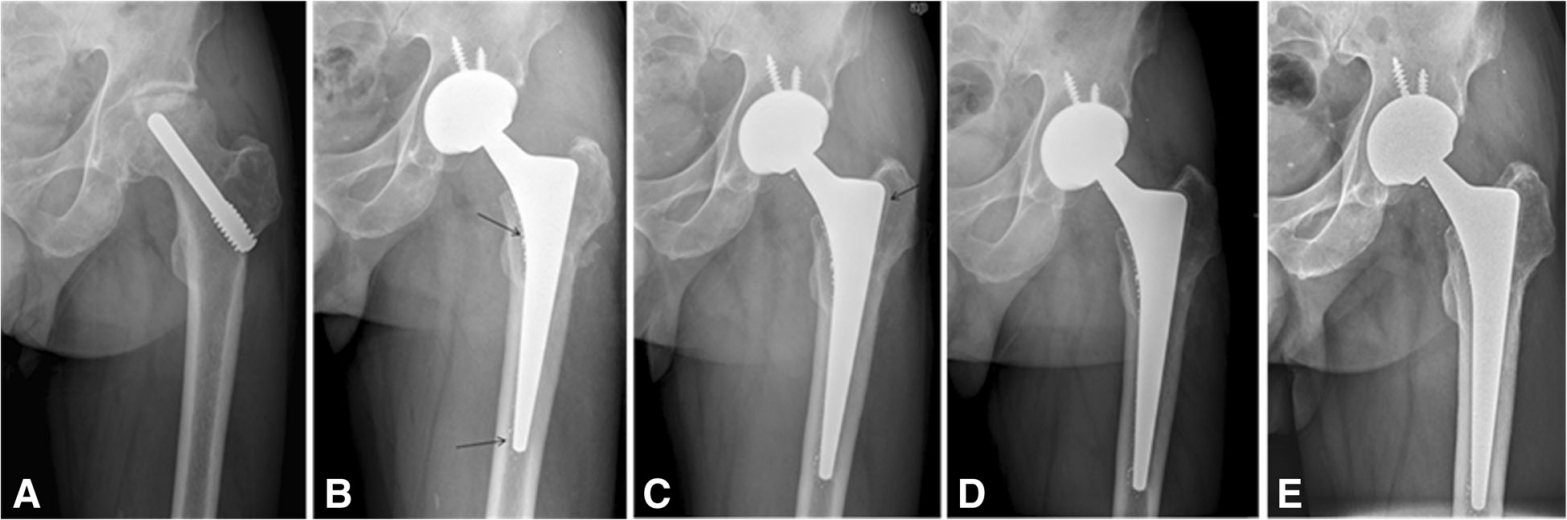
Radiographs of the left hip of a 46-year-old man with osteonecrosis of the femoral head (ONFH) after failure of the tantalum implant with conversion to total hip arthroplasty with ceramic-on-ceramic bearing. a Pre-operative radiograph. b Radiograph at 1-year, postoperatively, shows no osteolysis. Tantalum debris can be observed in the proximal, middle and distal regions (black arrows). c An anteroposterior view of the left hip at two years after total hip arthroplasty reveals a well-defined sclerotic edge near the medulla of the greater trochanter (black arrow). d Radiograph at four years, postoperatively, shows that the acetabular and femoral components are solidly fixed in a satisfactory position, with the radiolucencies in zones 1 and 7. e Radiograph at six years, postoperatively, shows no evidence of prosthesis loosening. The Harris score was 93 points at the last follow up
